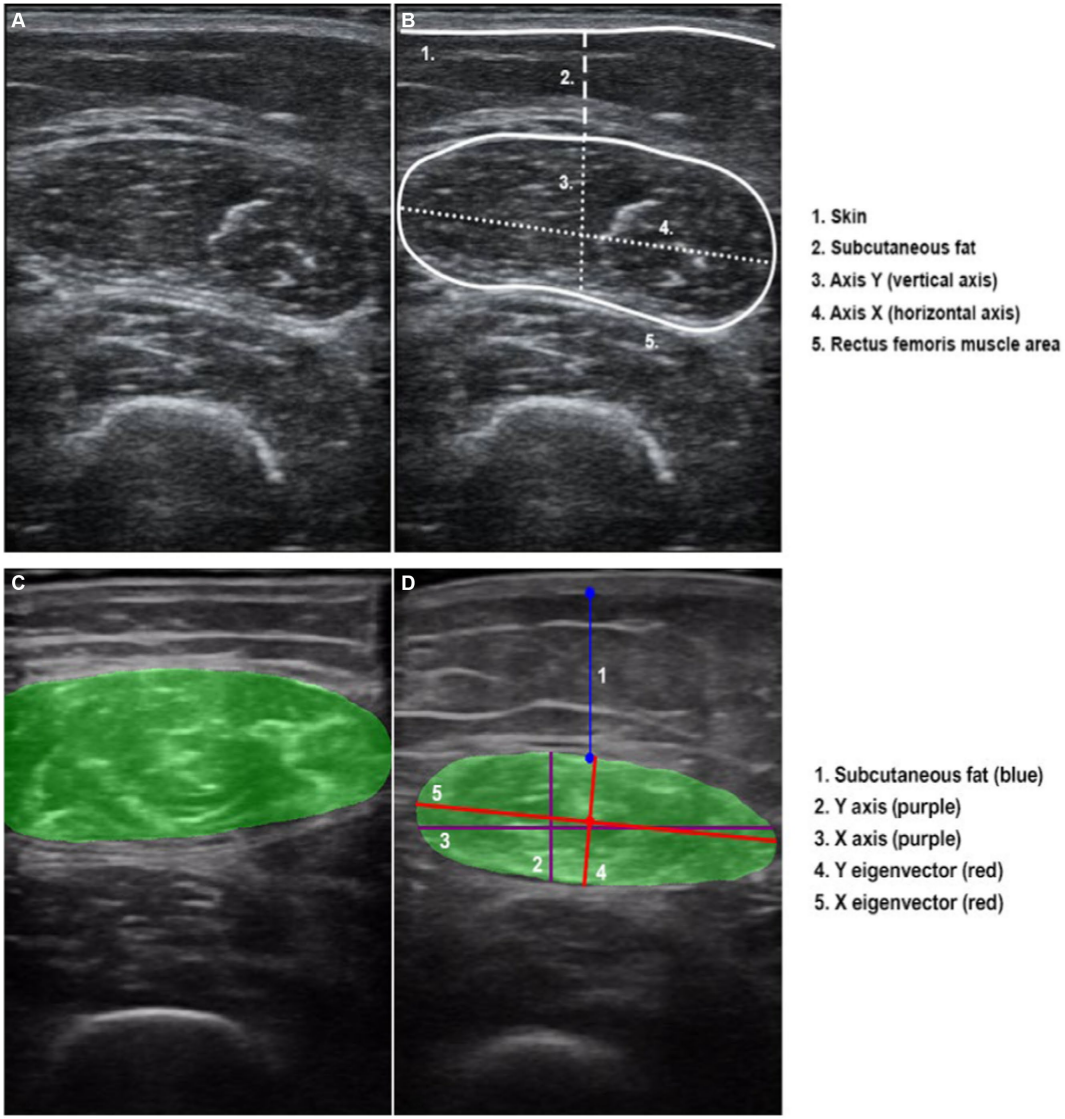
Ultrasound transversal section of rectus femoris. (A) Rectus femoris image without overflow. (B) Rectus femoris manual measures and scheme of the anatomical structures. (C) Image of rectus femoris with overflow. (D) Rectus femoris “Bat” measures, representing the “classic *X* and *Y* axes” and the reorientation using the automatic variables of the “*X* and *Y* eigenvector”.
